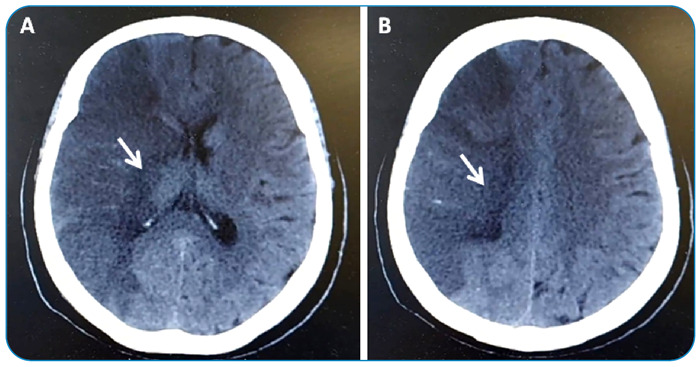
Ischemic stroke in the territory of the right middle cerebral artery (ischemic area indicated by white arrow) in a 54-year-old patient with Chagas disease. An echocardiogram showed reduced left ventricular ejection fraction with thrombus at apical aneurysm, classifying the stroke as cardioembolic.
